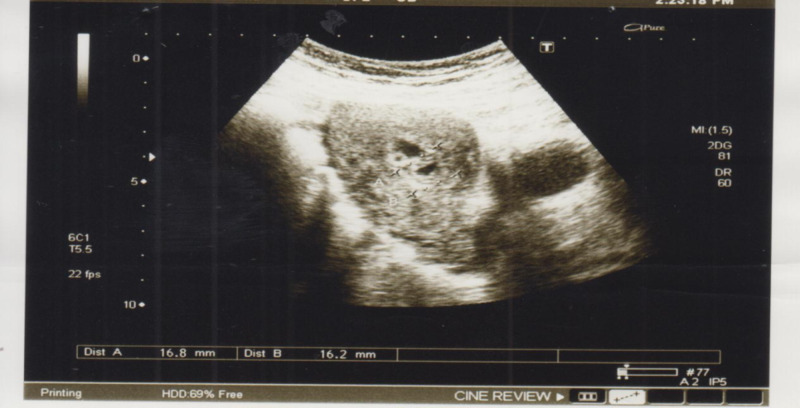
Pelvic ultrasound with thick endometrium and increased vascularity
